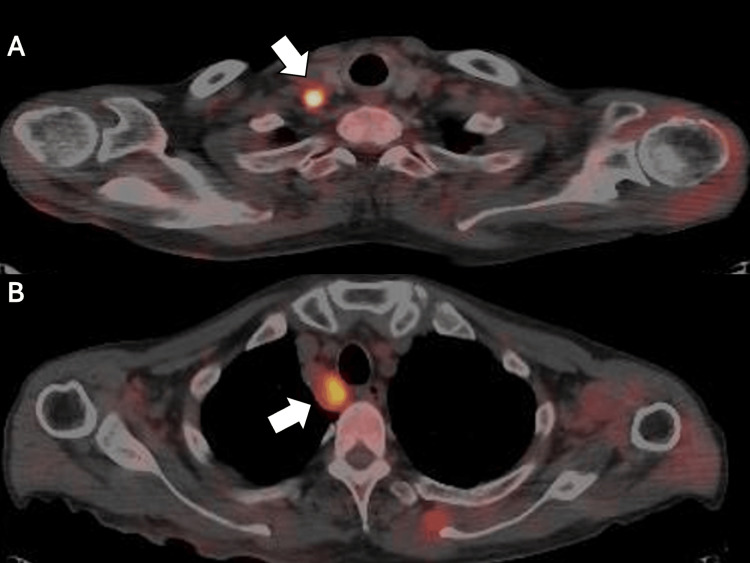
Positron emission tomography-computed tomography demonstrating the uptake in the right supraclavicular (A) and right paratracheal (B) lymph nodes (white arrows).
